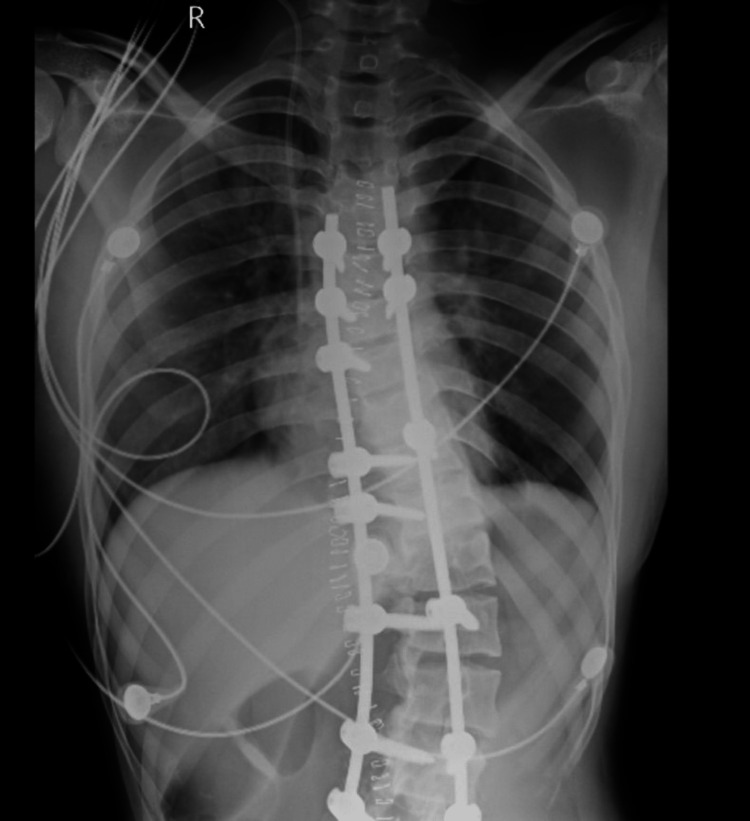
Postoperative chest X-ray showing a central venous catheter in the right jugular vein.
